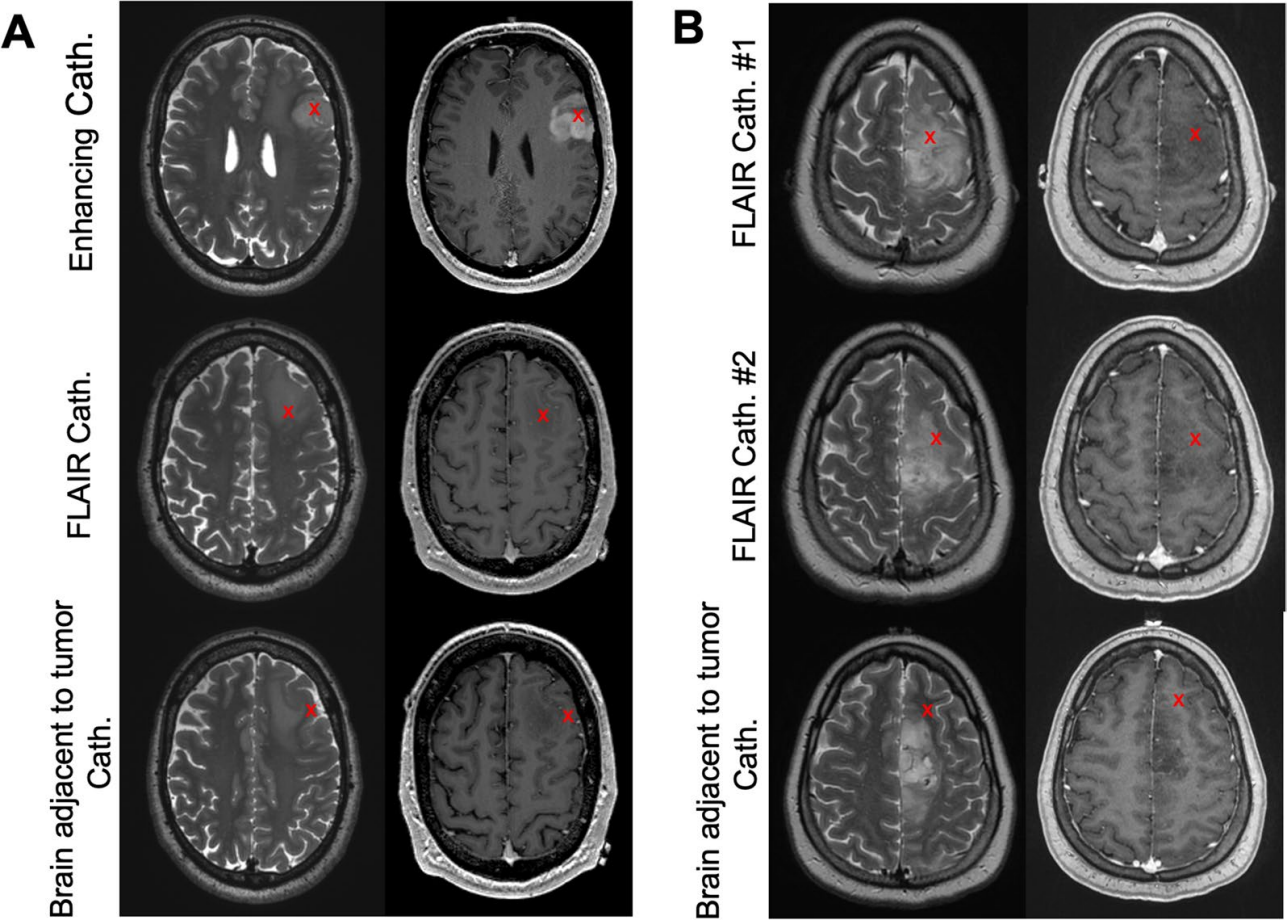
Pre-operative magnetic resonance imaging (MRI) was utilized to define microdialysis catheter placement in radiographically diverse regions. A In patients with contrast-enhancing tumors, microdialysis catheters were placed in enhancing and non-enhancing tumor, as well as brain adjacent to tumor. B In patients with non-contrast enhancing tumors, two microdialysis catheters were placed in non-enhancing tumor and one in brain adjacent to tumor. Left: T2 FLAIR; right: T1-weighted post-contrast sequence; X indicates location of catheter placement
